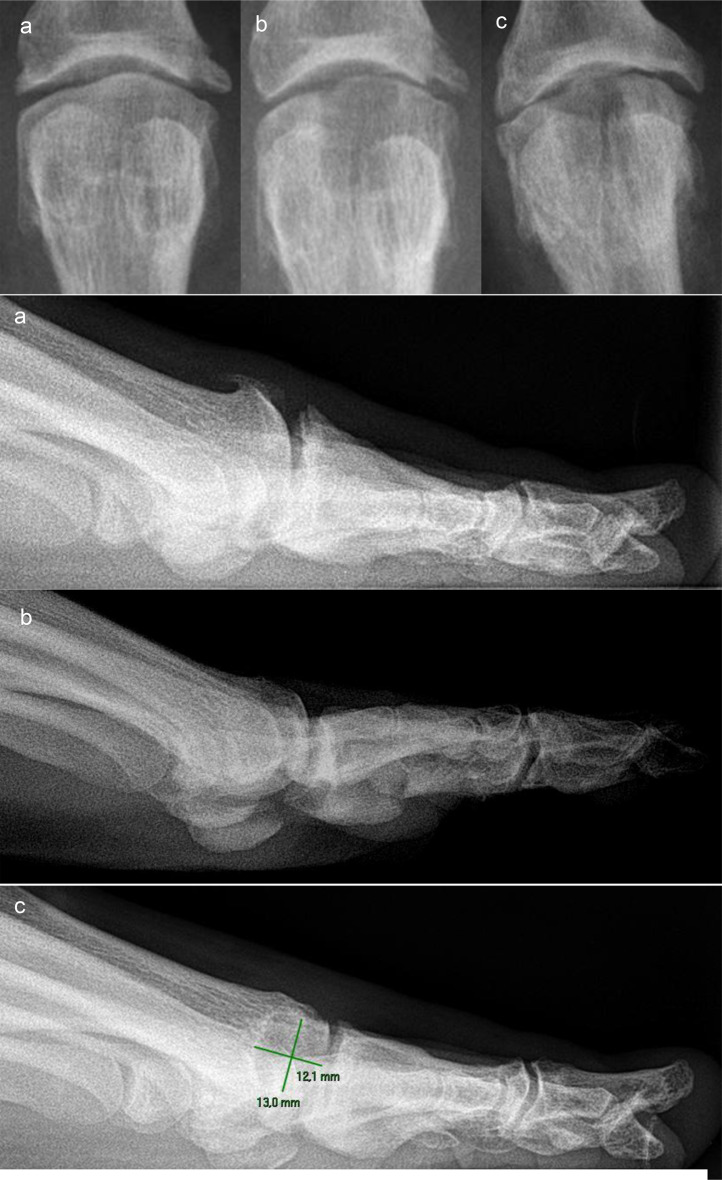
Case of a satisfactory clinical outcome a: Preoperative condition. b: Radiological control 6 weeks postoperatively: no abnormalities and satisfactory joint space. c: Follow-up, 23 months postoperatively: Joint space now reduced to some considerable extent, but mobility satisfactory. No reaction of the surrounding tissue in the anteroposterior projection, but enlargement of the implant site in the lateral radiograph. The patient is completely pain-free
